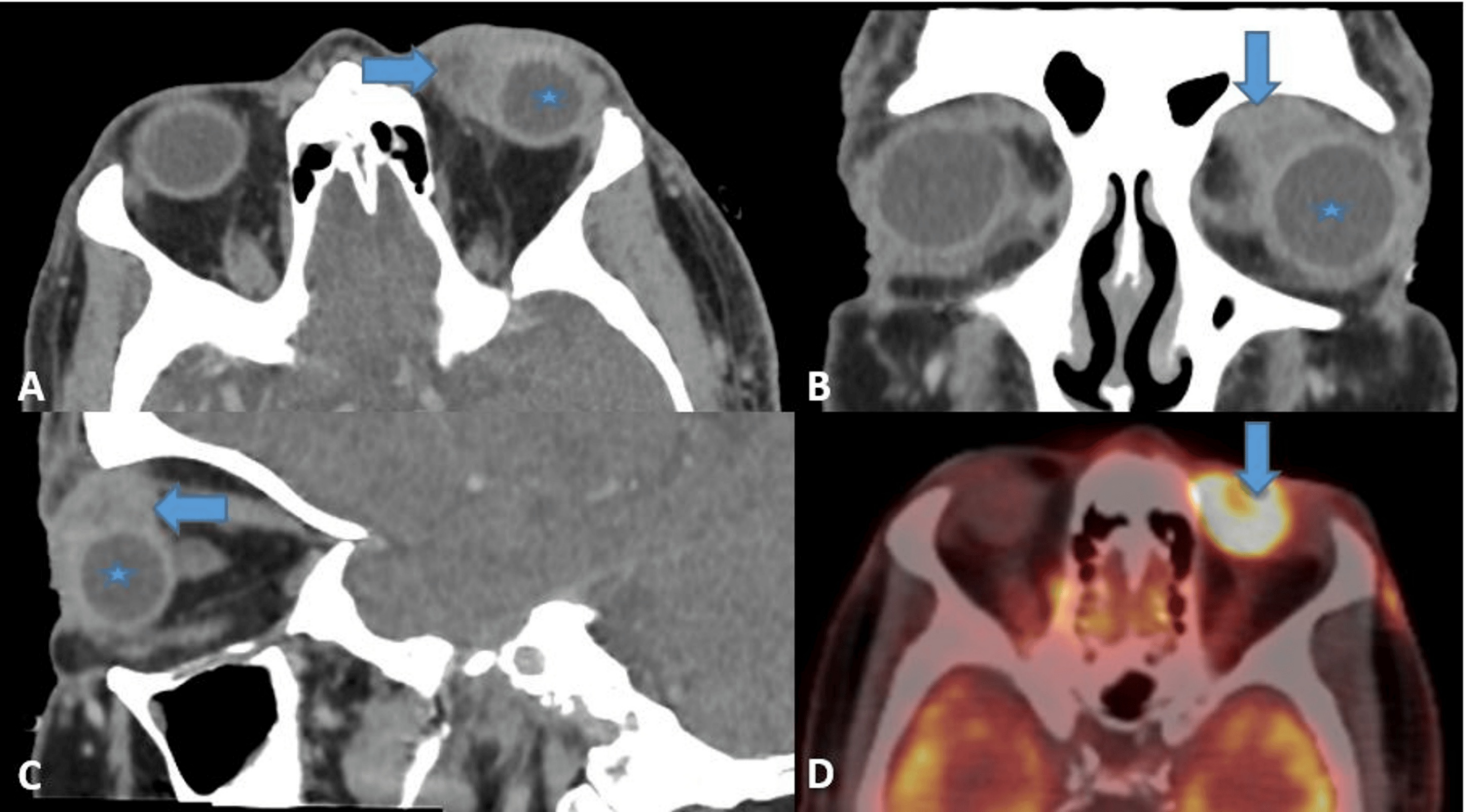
Imaging findings. Contrast-enhanced computed tomography of the orbits with soft tissue reconstruction: axial view (A), coronal view (B), sagittal view (C). Axial view of 18F-FDG PET-CT scan (D). A space-occupying lesion with ill-defined margins (arrows) is observed in the superomedial angle of the left orbit, showing mild peripheral enhancement. The globe is not involved (*). The lesion is hypermetabolic on PET-CT, with a maximum standardized uptake value (SUVmax) of 11.21. FDG: fluorodeoxyglucose
